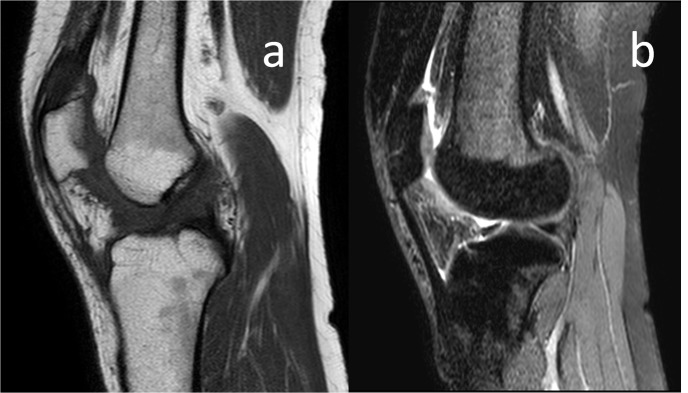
Final control MRI examination of the patient shows no signs of recurrence. MRI: magnetic resonance imaging
